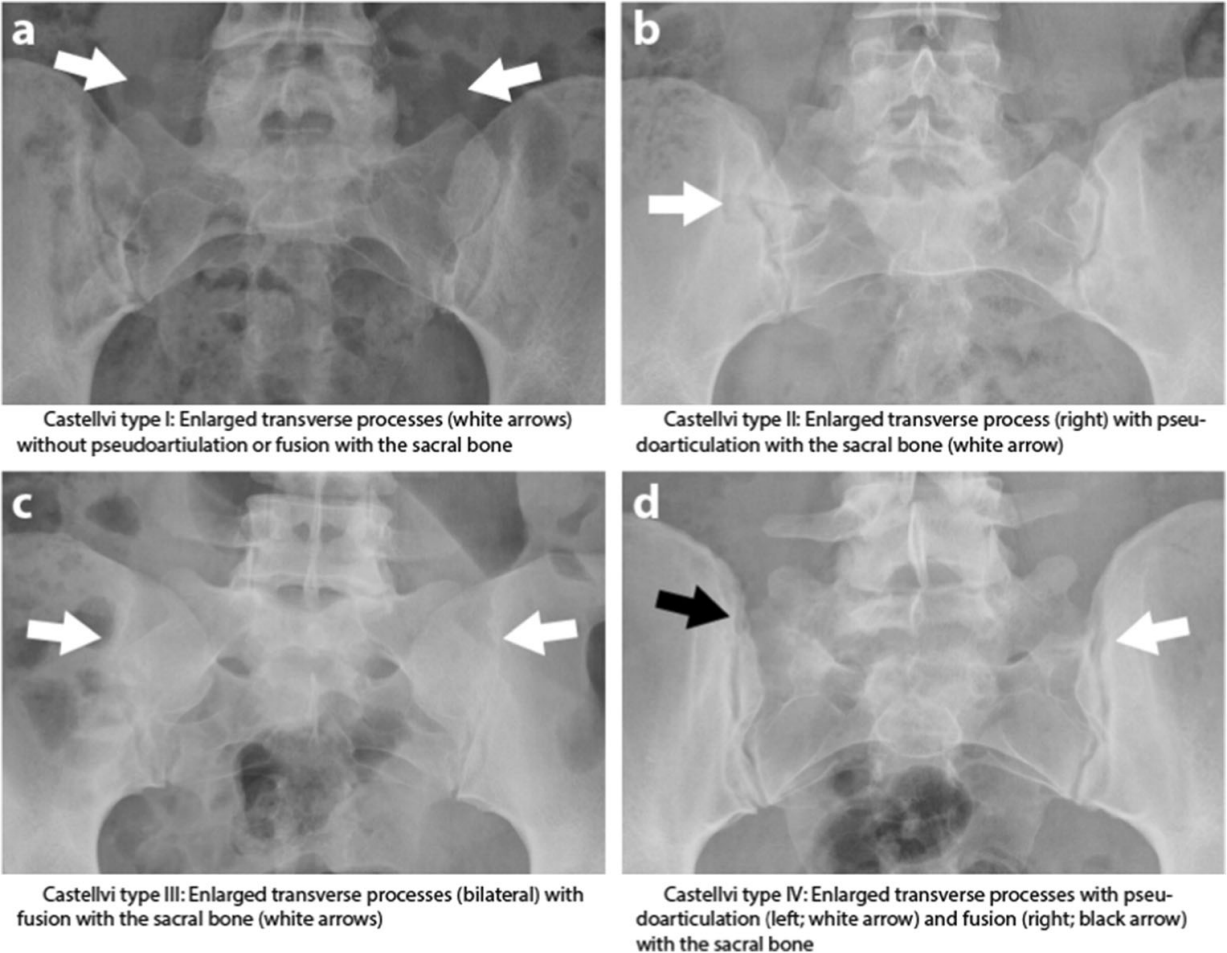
Radiographs of the Castellvi classification of lumbosacral transitional vertebra. a Type I: enlarged transverse processes (*white arrows*) without pseudoarticulation or fusion with the sacral bone. b Type II: enlarged transverse process (*right*) with pseudoarticulation with the sacral bone (*white arrow*). c Type III: enlarged transverse processes (bilateral) with fusion with the sacral bone (*white arrows*). d Type IV: enlarged transverse processes with pseudoarticulation (*left*; *white arrow*) and fusion (*right*; *black arrow*) with the sacral bone
